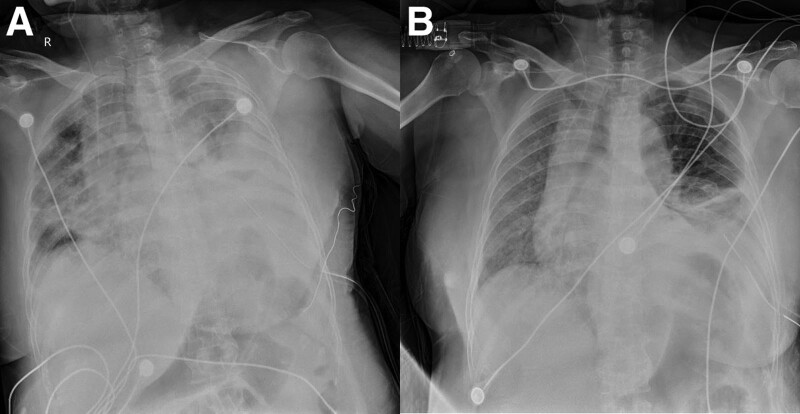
Chest radiographs of the patient. Initial supine chest radiography obtained on presentation to the ICU (A) shows pulmonary edema, left pleural effusion. A supine anteroposterior portable chest radiograph obtained 3 d after presentation to the ICU (B) shows left atelectasis and pleural effusion and right alveolar infiltrate. ICU = intensive care unit.
